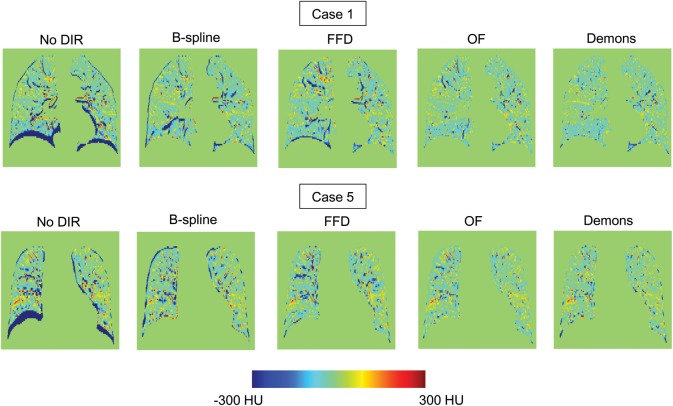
Difference images for registration of inhalation-to-exhalation 4D CT volumes for Case 1 and Case 5. No DIR = no deformable image registration, B-spline = deformable image registration with B-spline, FFD = deformable image registration with free-form deformation, OF = deformable image registration with Horn–Schunk optical flow, Demons = deformable image registration with Demons.
